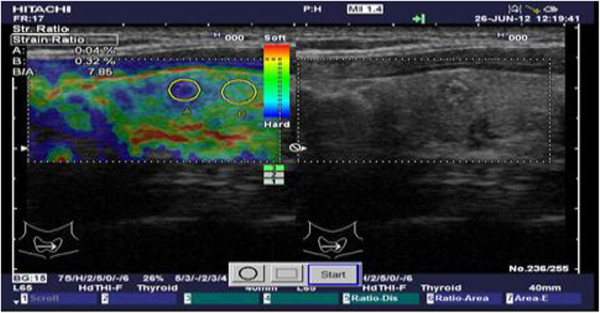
Elastosonographic and ultrasound images of the thyroid nodule.
